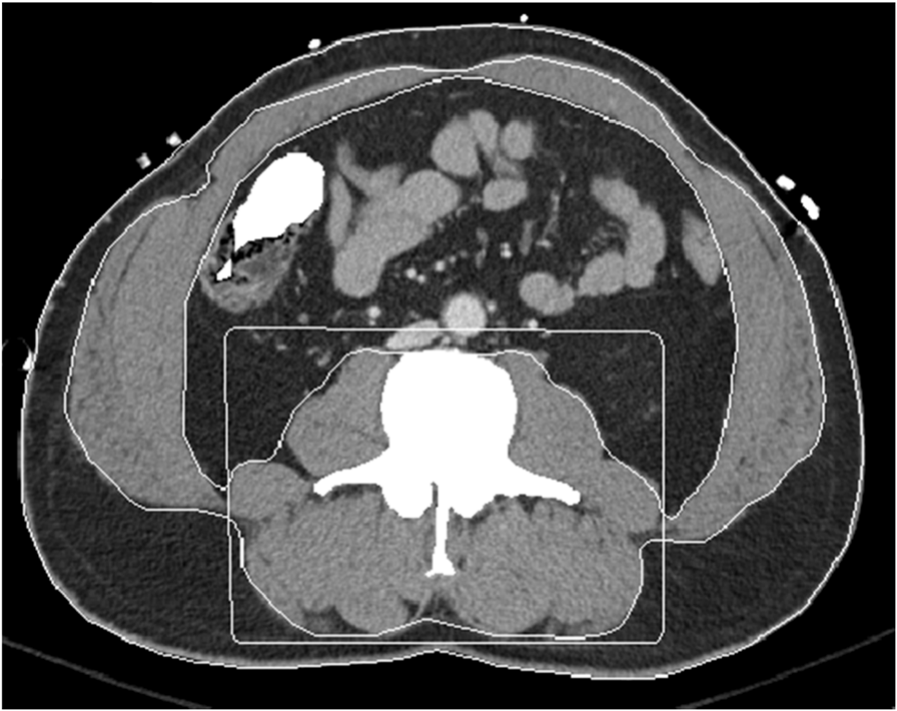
Segmentation of a computed tomography image of a 55-year-old nonsarcopenic male with a skeletal muscle index of 72 cm^2^/m^2^
